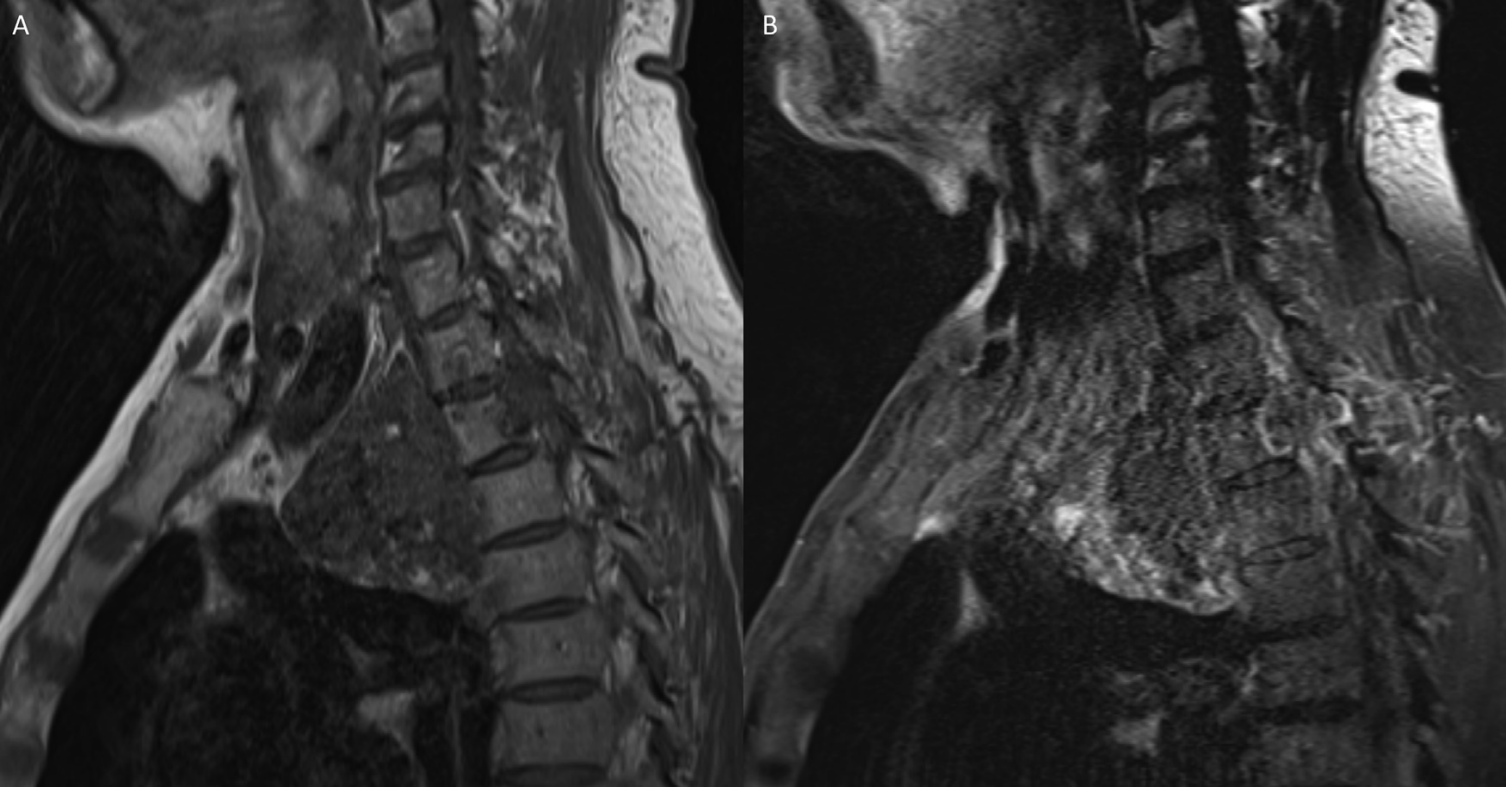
MRI brachial plexus protocol, T1 coronal (A) and T1 post gadolinium coronal (B) demonstrates extension of this mass into the right T1-T2 and T2-T3 neural foramina (not included in the figure) and T1-T2 right lateral epidural space, with mild mass effect on the thecal sac
